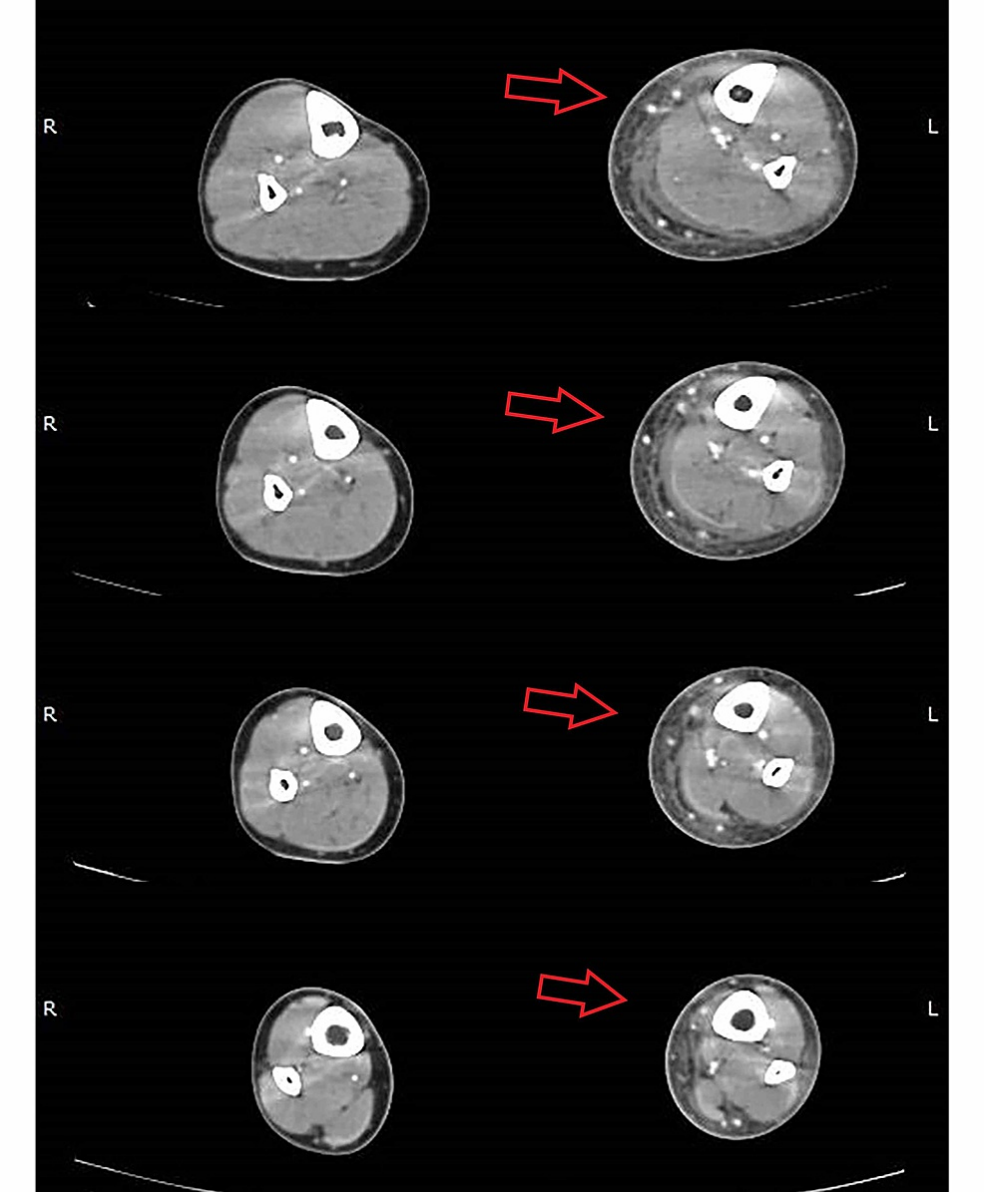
Arterial-phase CT axial images show circumferential edema of subcutaneous tissue, thickening of the crural fascia, and fluid flap on the medial side of leg, close to gastrocnemius muscle belly. CT, computed tomography
